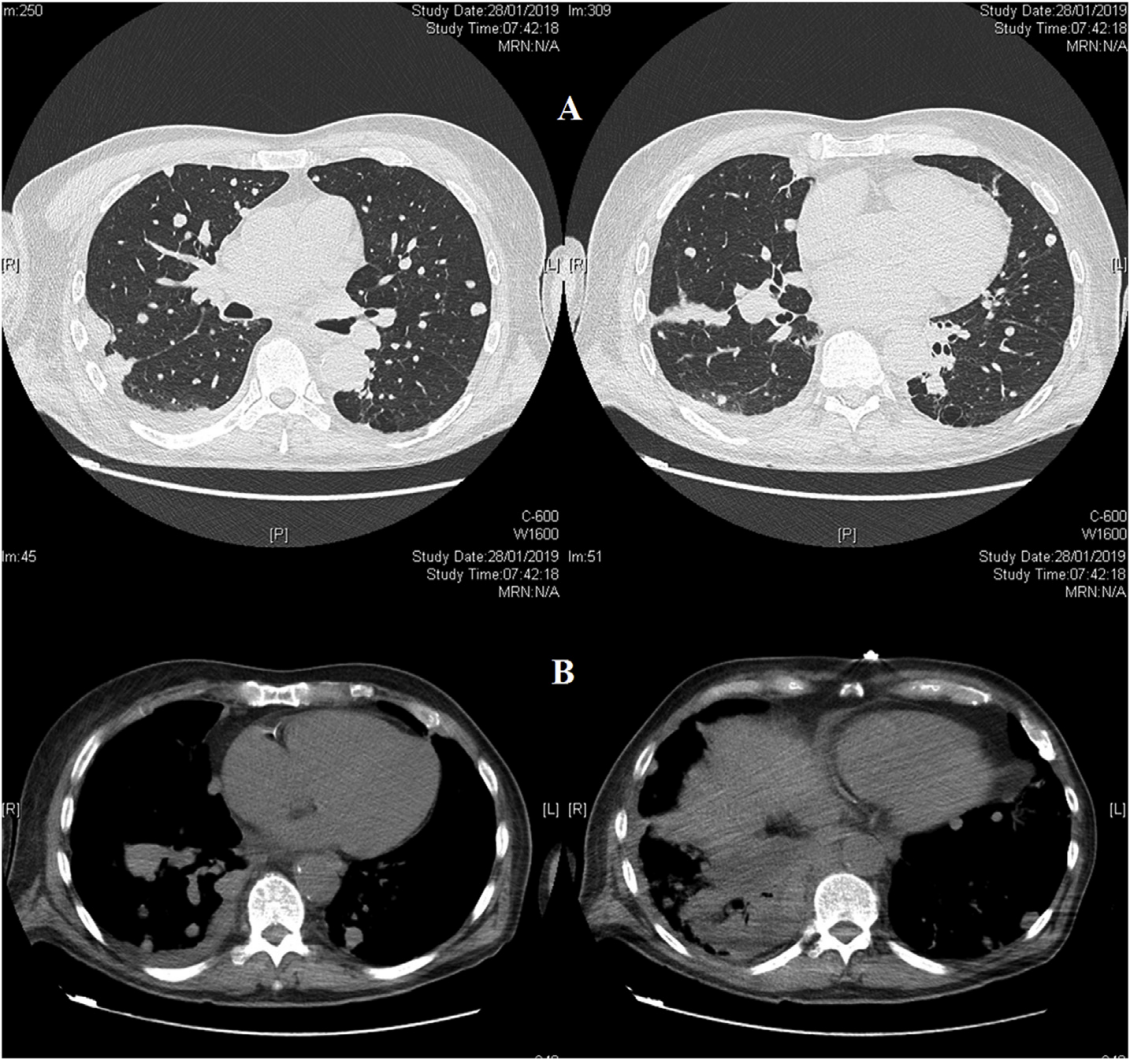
Patient's CT scan with multiple metastatic lung nodules (A) and irregular pleural thickening.
